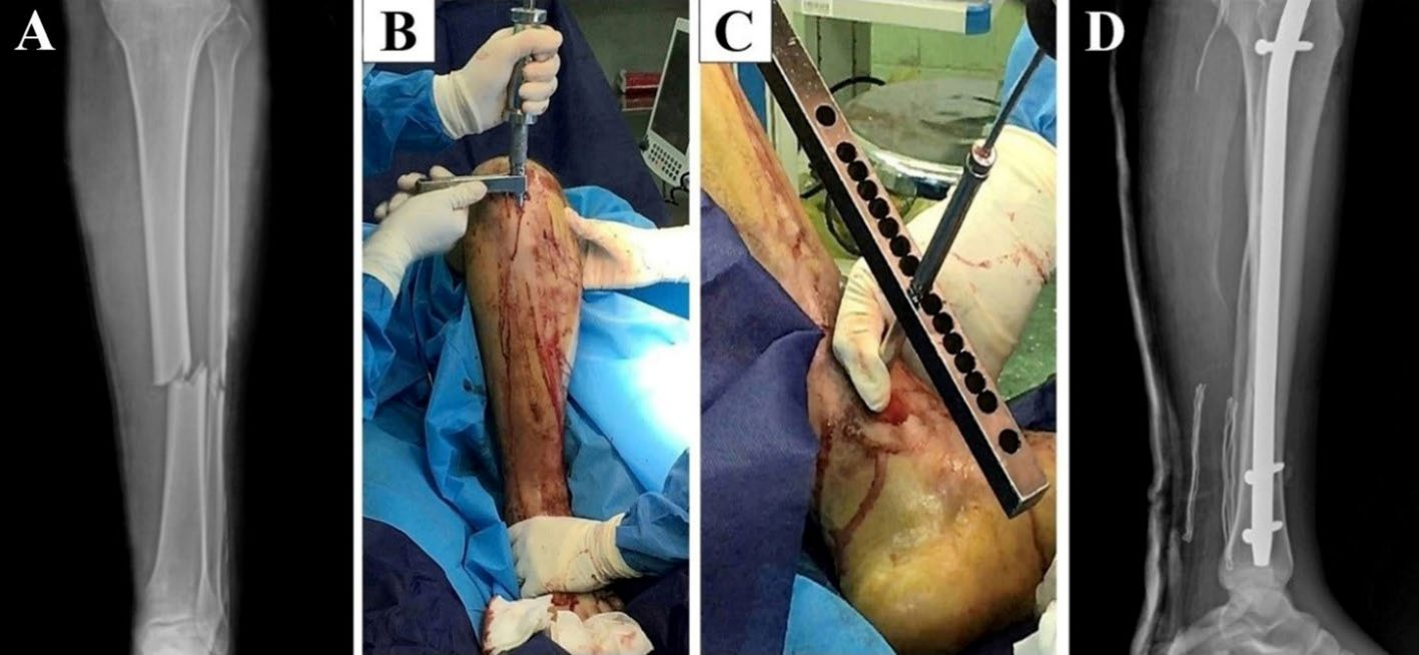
Interlocking of the intramedullary nail by the TAD, (A) an X-ray image of the patient's left foot before the surgery, (B) Inserting the IMN inside the tibia bone using the hammer, (C) Distal interlocking of the IMN by the TAD, (D) The X-ray image of the patient's left foot one day after surgery.
